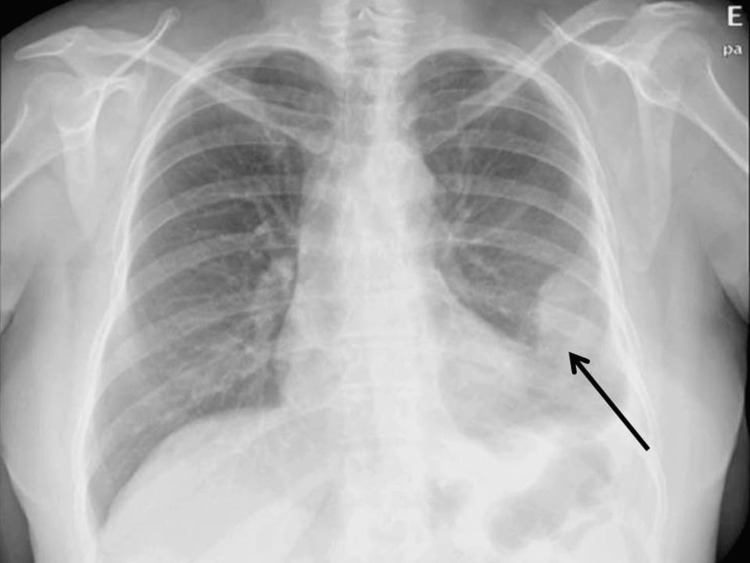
Chest X-ray - May 2021, with hypodensity at left lung base (arrow)
